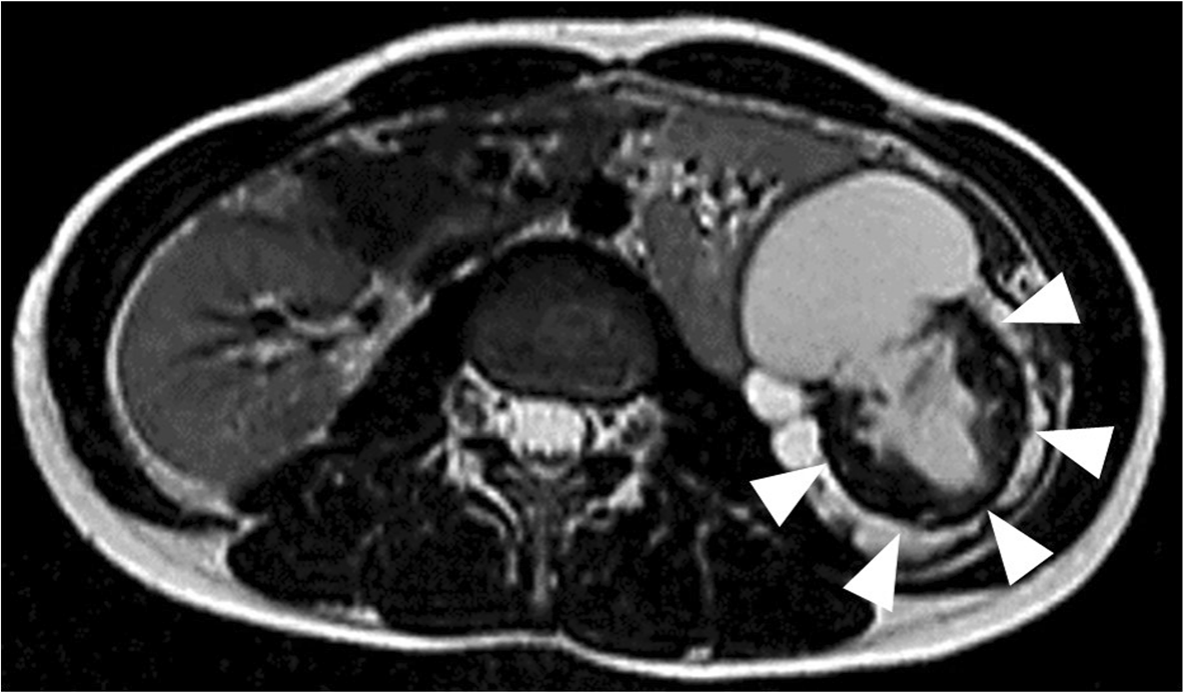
Abdominal magnetic resonance imaging. A T2-weighted magnetic resonance image showing a high-intensity tumor (arrowheads).
